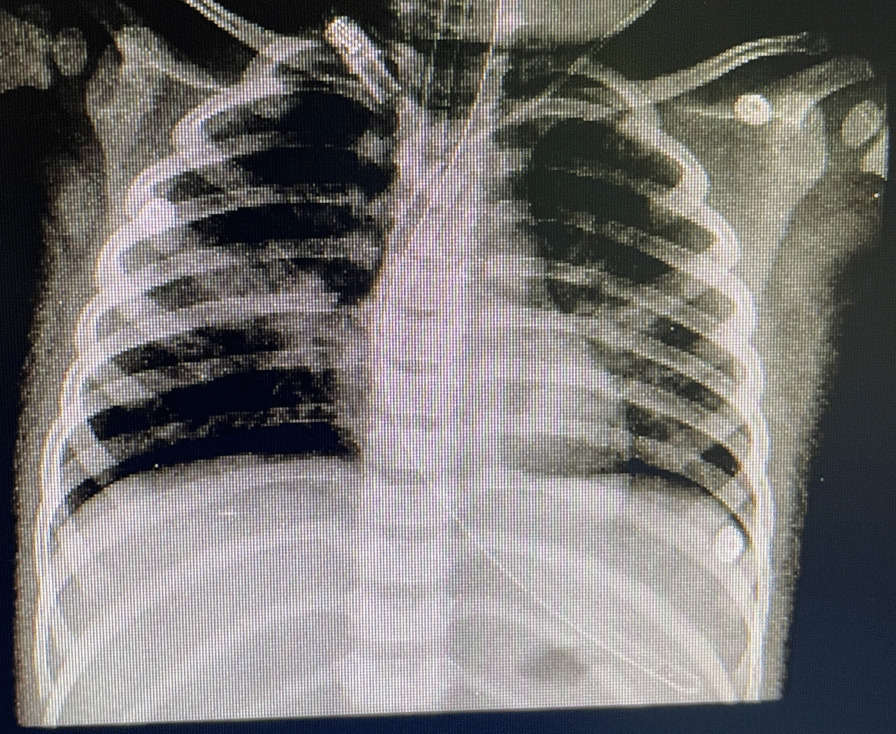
A chest radiograph showing consolidation in the middle zone of the right lung during a lung radiography scan
